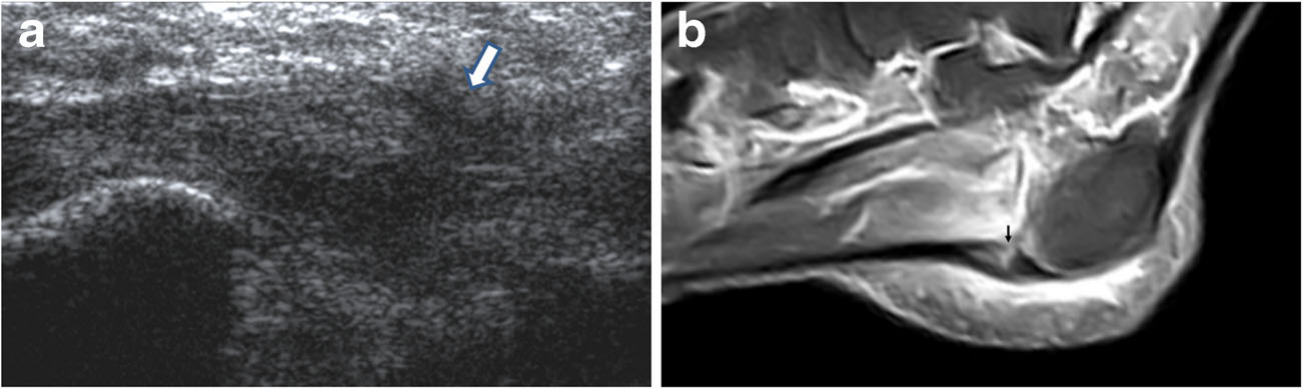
Plantar fascia rupture. On ultrasound, a tear in the PF (*arrow*) is shown; the PF is hypoechoic and thickened as a result of previous plantar fasciitis treated with local injections (a). MRI confirms PF rupture (*arrow*) and highlights marked oedema of soft tissues (b)
